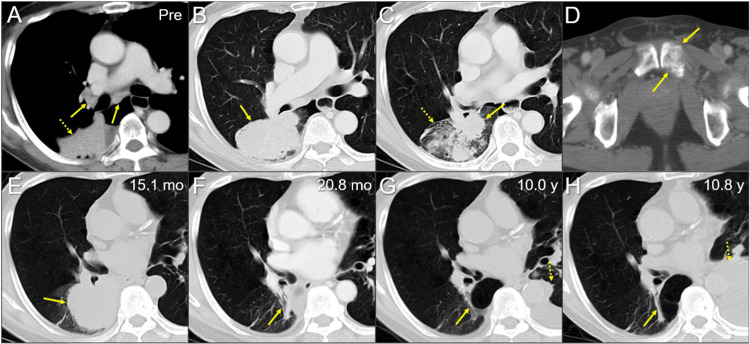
Contrast-enhanced (CE) and non-CE thoracic and pelvic CT images The images show axial contrast-enhanced (CE) CT images (A-D, F); non-CE CT images (E, G, H); thorax (A-C, E-H); pelvis (D); soft tissue window (A); lung window (B, C, E-H); bone window (D); before first-line chemotherapy (Pre) (A-D); at 15.1 months (mo) after the first-line chemotherapy (E); at 20.8 months (F); at 10.0 years (y) (G); and at 10.8 years (H) (A-H) These images are shown at the same magnification and coordinates under co-registration and fusions. (A-C) Multiple lymph node swelling (arrows in A) in the right hilar and subcarinal regions and a well-demarcated mass lesion (arrows in B, C) associated with secondary changes due to partial atelectasis (dashed arrow in A) and pneumonitis (dashed arrow in C) in the right lower lobe. (D) An osteolytic lesion with a pathological fracture in the left pubic bone (arrows in D). (E) At 15.1 months, the perihilar lesion (arrow in E) was refractory to fourth-line chemotherapy. (F) At 20.8 months (after six courses of fifth-line chemotherapy), the lesion regressed remarkably, only leaving scar tissue (arrow in F). (G, H) At 10.0 to 10.8 years, the lesion remained in complete regression (arrows in G, H), while pleural dissemination (dashed arrows in G, H) progressed in the contralateral thorax CT: computed tomography
